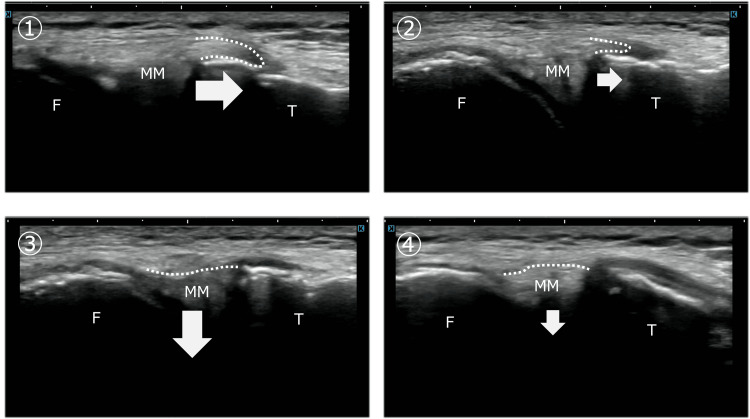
Dynamic movement of the medial meniscus during tibial rotation: comparison between the unaffected and affected sides on ultrasonography Ultrasonography was performed with the knee in slight flexion while tibial internal and external rotation was applied to assess medial meniscus (MM) movement associated with changes in medial collateral ligament (MCL) tension. Panels ① and ② represent tibial internal rotation on the unaffected and affected sides, respectively. On the unaffected side, the MM moved smoothly toward the extracapsular side during internal rotation, whereas on the affected side, the magnitude of MM movement was reduced. Panels ③ and ④ represent tibial external rotation on the unaffected and affected sides, respectively. On the unaffected side, the MM entered the medial joint space sufficiently during external rotation; however, on the affected side, inward excursion of the MM was limited. These findings indicate restricted MM dynamics on the affected side during both internal and external tibial rotation.
